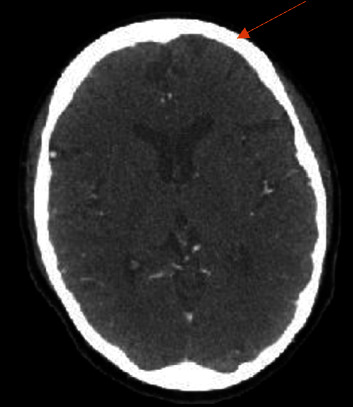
Computed tomography angiography head and neck of the patient showing ischemia of the brain. This image is of a computed tomography angiography head and neck. The orange arrow notes mild hypoattenuation in the high left frontal convexity.
